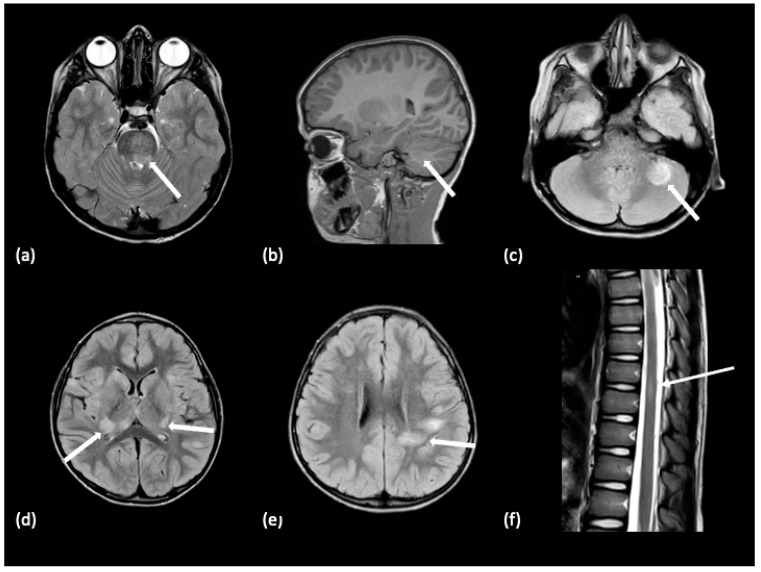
A 7-year-old male presented with an acute history of fever, ataxia, and dysarthria. Brain MRI demonstrated multiple hyperintense signals on T2/FLAIR sequences that displayed faint hypointense signals on T1-weighted sequences, without diffusion restriction, abnormal contrast agent enhancement or mass effect. Lesions are noted with white arrows. (a) Lesions within the pons and cerebral peduncle are hyperintense on an axial T2-weighted image. (b) Sagittal T1-weighted and (c) axial FLAIR feature a lesion in the left cerebellar hemisphere. (d,e) Axial FLAIR images demonstrate bilateral thalamic and left centrum semiovale lesions, respectively. (f) An additional ill-defined, T2-mildly hyperintense lesion was noted at the T9–10 of the spinal cord. This lesion was without abnormal enhancement on T1-weighted imaging. These imaging features supported the diagnosis of acute demyelinating encephalomyelitis (ADEM).
